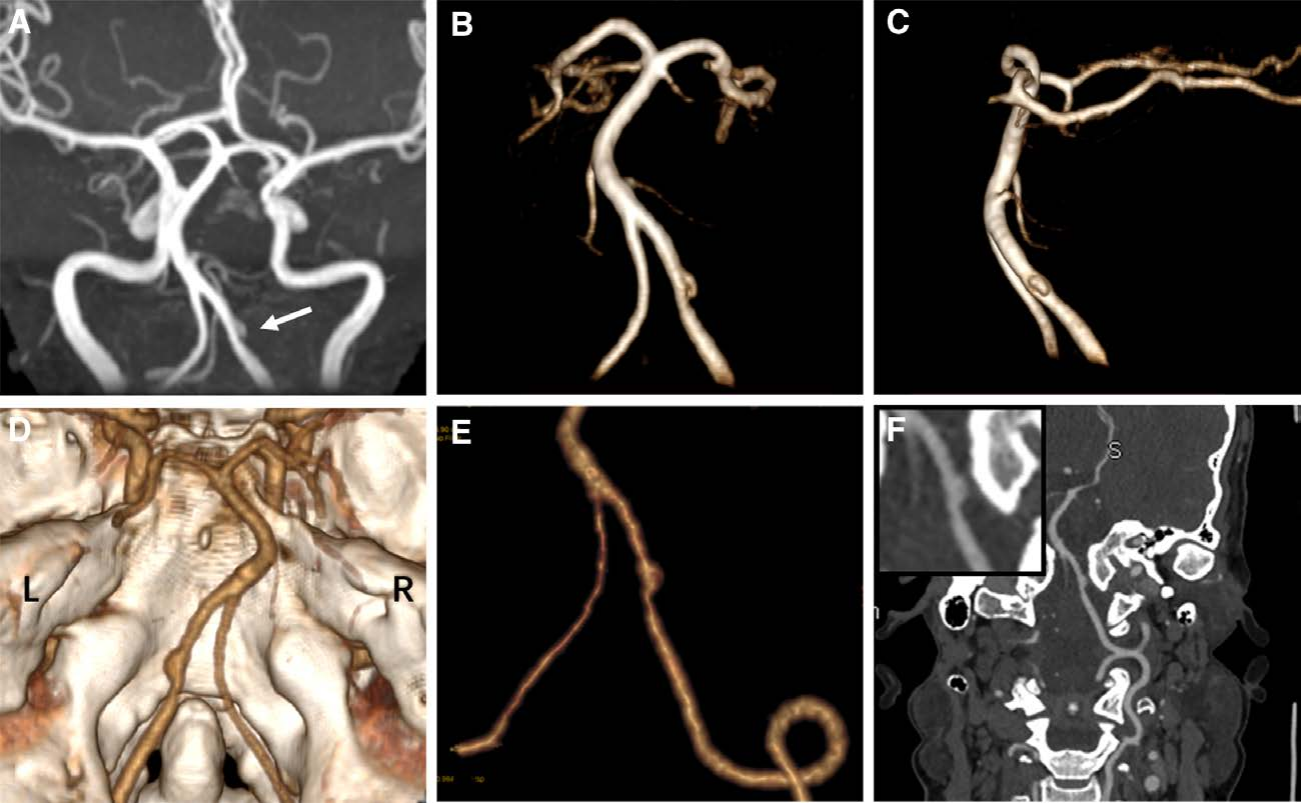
Maximum intensity projection (MIP) time-of-flight magnetic resonance angiography (TOF MRA) (A), anterior (B), and lateral projection (C) of 3-dimensional TOF MRA reconstruction image, 3-dimensional reconstruction of computed tomography angiography (CTA) (D, E), and MIP image (F) revealed a left vertebral artery dissection-like aneurysm (arrow) (4.5 × 2.0 × 2.5 mm in size) with mild stenosis of the proximal parent artery (40%). CTA = computed tomography angiography, MIP = maximum intensity projection, TOF MRA = time-of-flight magnetic resonance angiography.
